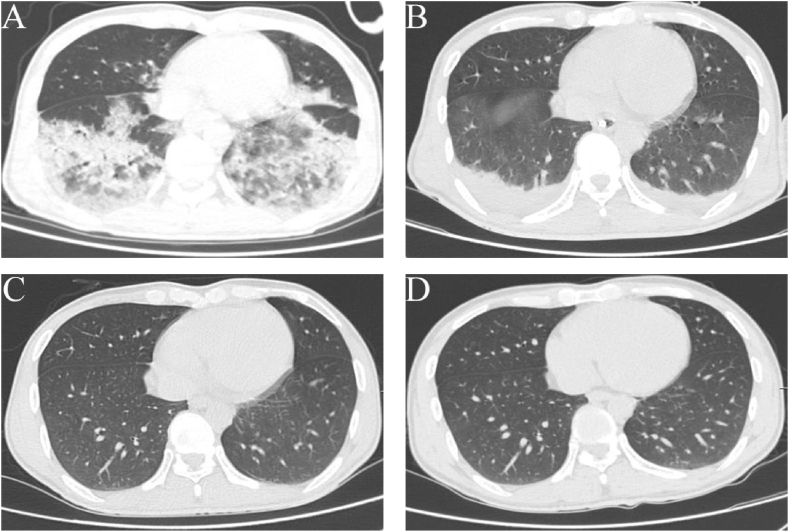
Chest CT imaging of the patient. A. Chest CT scan at the time of presentation revealed diffuse patchy shadows in both lungs; B. A chest CT scan prior to tracheal intubation and extubation indicated that the patchy shadows in both lungs had greatly diminished; C. A chest CT scan prior to the patient's hospital discharge revealed that the patchy shadows in both lungs had significantly improved; D. A chest CT scan one month later revealed that there were no significant patchy shadows in either lung. CT, computed tomography.
